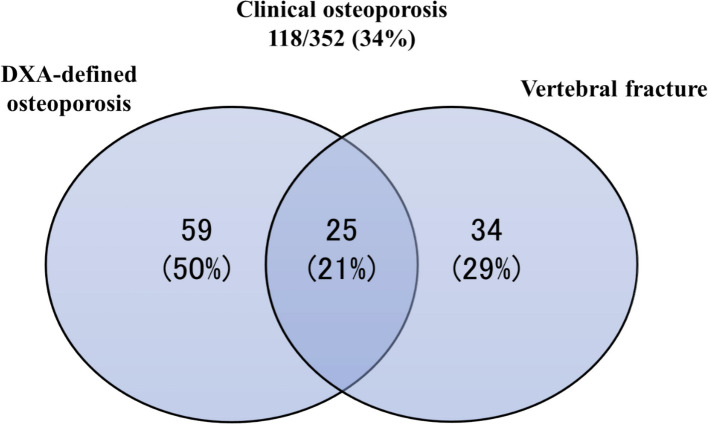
Overlap between dual-energy X-ray absorptiometry (DXA)-defined osteoporosis and vertebral fractures. Clinical osteoporosis was defined as DXA-defined osteoporosis or a prevalent vertebral fracture (VF). Numbers indicate the number of patients in each category, and percentages are calculated relative to the 118 patients with clinical osteoporosis. Of the 352 patients, 59 had DXA-defined osteoporosis alone, 25 had both DXA-defined osteoporosis and VFs, and 34 had VFs without DXA-defined osteoporosis
